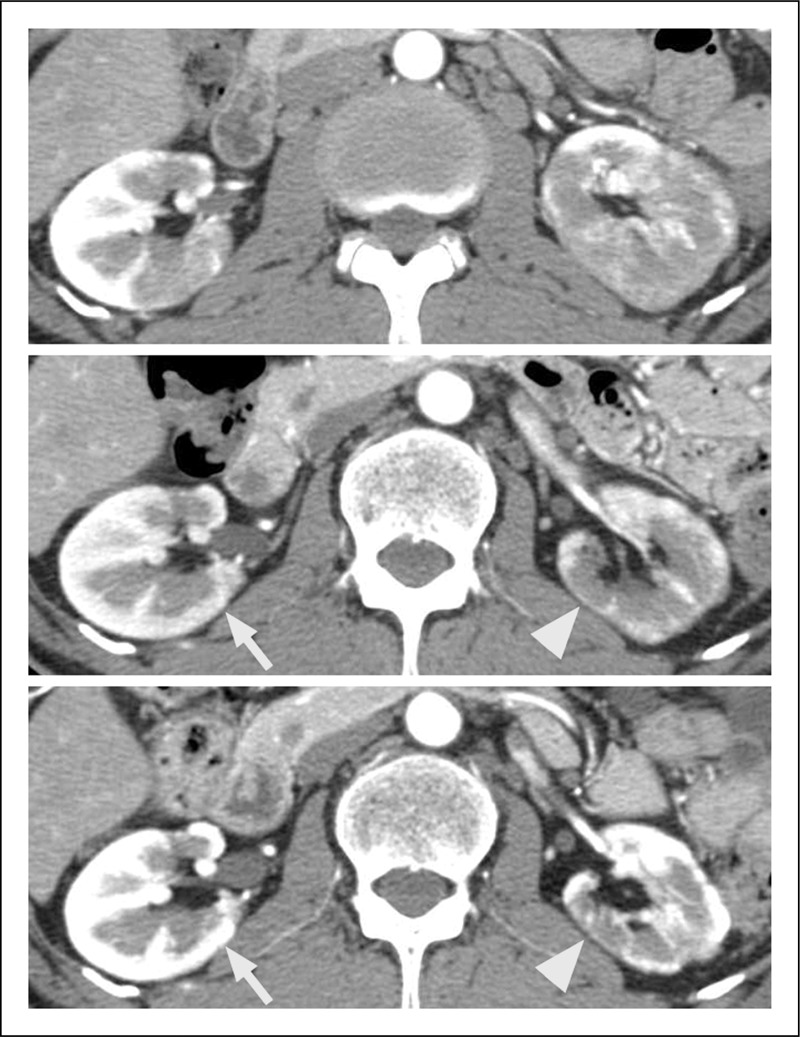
Longitudinal changes of imaging findings during corticosteroid therapy. Upper: Contrast-enhanced computed tomography (CT) scan before corticosteroid therapy shows multiple low-density lesions in the bilateral kidneys in a patient with IgG4-RKD. Middle: Two months after starting steroid therapy, contrast-enhanced CT scan shows complete recovery and disappearance of low-density lesions without atrophy in some areas of the kidney (arrow), while atrophic scarring starts to appear in other areas (arrowhead). Lower: Six years after therapy and still under steroid maintenance therapy, the area that showed early recovery maintains its normal appearance without atrophy (arrow), but the area where atrophic scarring started to appear shows progressive scarring with decreased enhancement (arrowhead) on contrast-enhanced CT scan.
